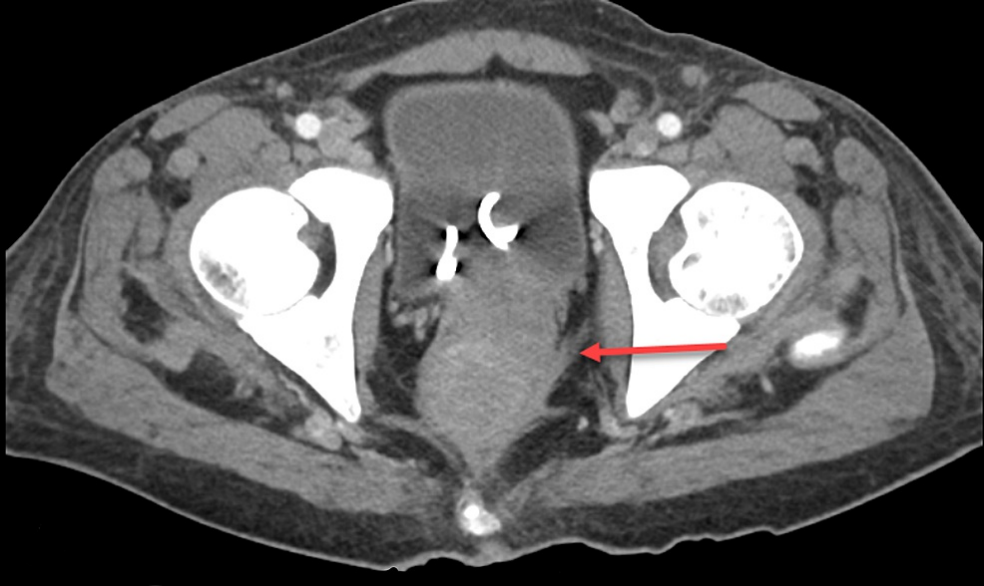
CT cross-sectional view: red arrow demonstrates infiltrative soft tissue leading to large bowel and rectum obstruction.
